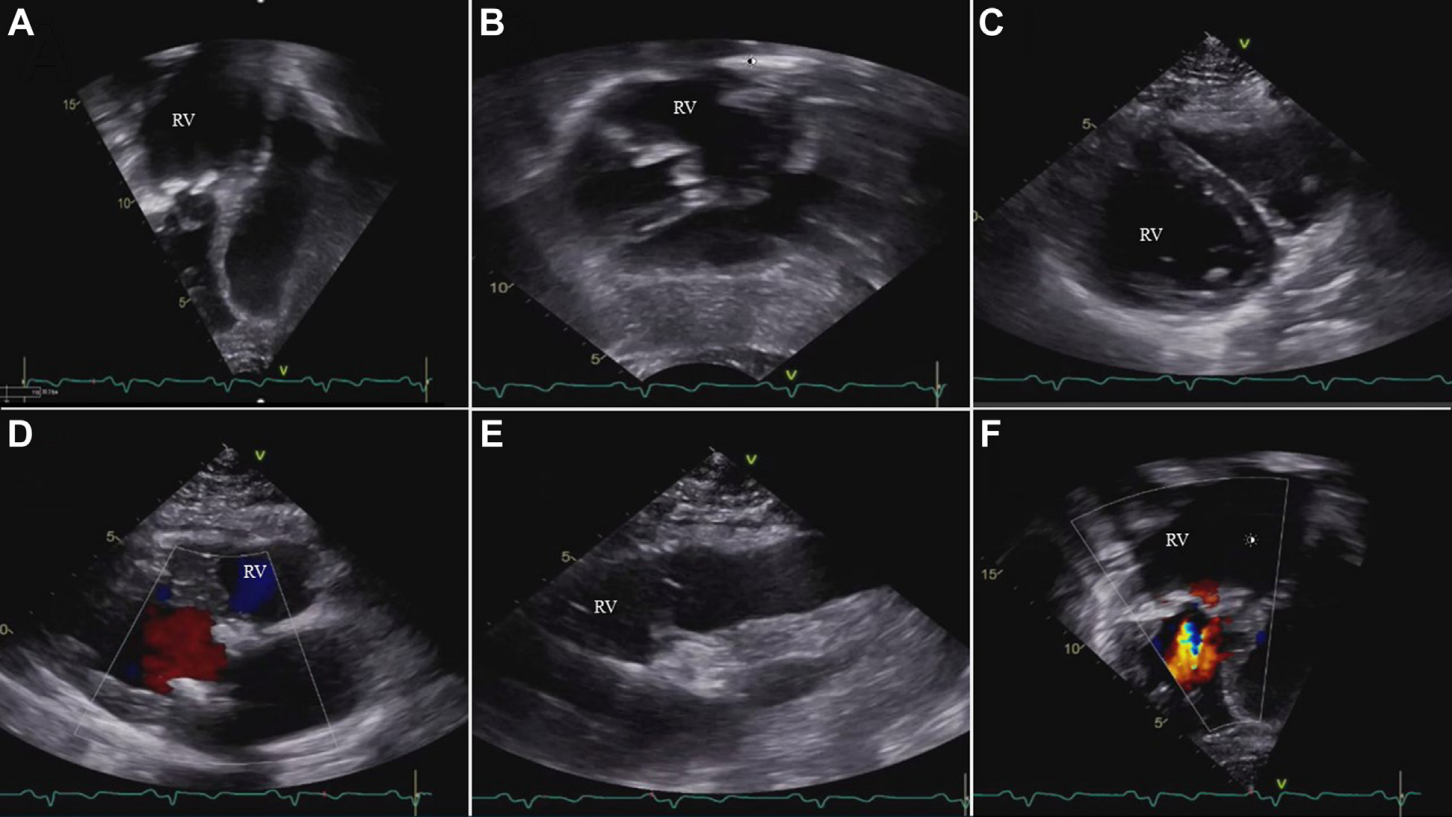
Transthoracic Echocardiography: Multiple Views The following views demonstrate systemic right ventricular hypertrophy, mild to moderate systolic dysfunction, and a stenotic appearing tricuspid valve. (A) 4-chamber view. (B) Subcostal view. (C) Short-axis view. (D) Long-axis view. (E) Long-axis aorta view. (F) Turbulent flow in the 4-chamber view. RV = right ventricle.
